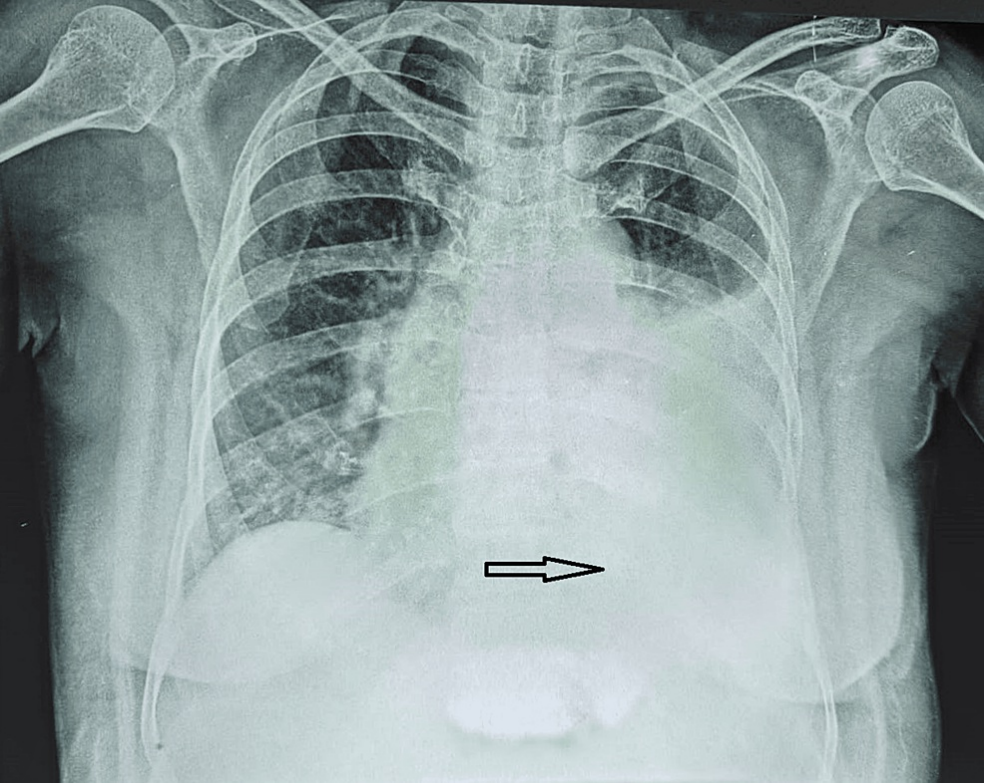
Chest radiograph shows sided pleural effusion
